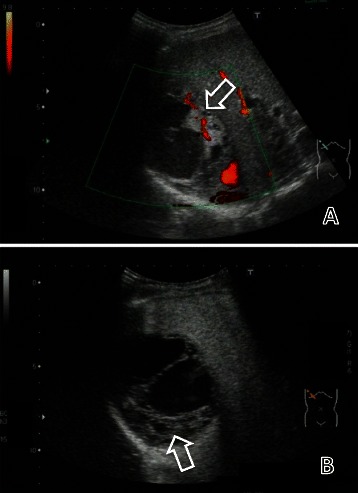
Color Doppler and contrast-enhanced ultrasonography showing the multilocular cystic liver mass. Color Doppler ultrasonography (A) and vascular phase contrast-enhanced ultrasonography using Sonazoid (92 seconds after bolus injection) (B) showed the multilocular cystic mass consisted of thickened irregular septa and nodal walls with blood flow.
